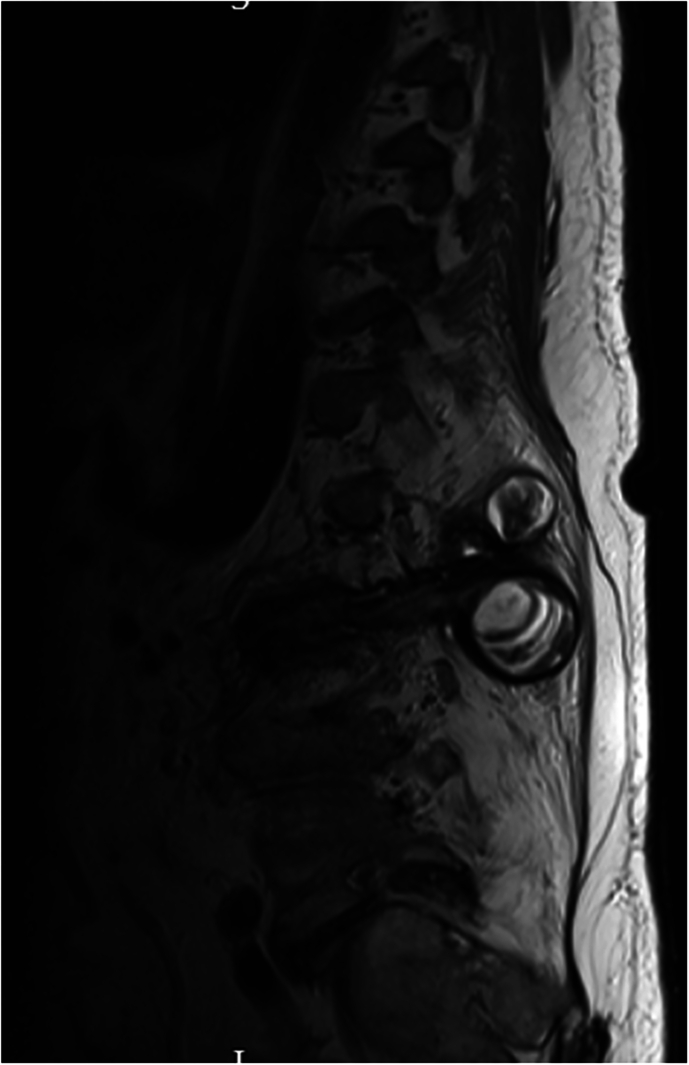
Sagittal view of lumbar MRI: L2/L3 irregular and displaced spinal pseudoarthrosis above the fusion segment. Soft tissue material interposed locally with posterior parents paraspinal fluid collection measuring 7.5 cm.
